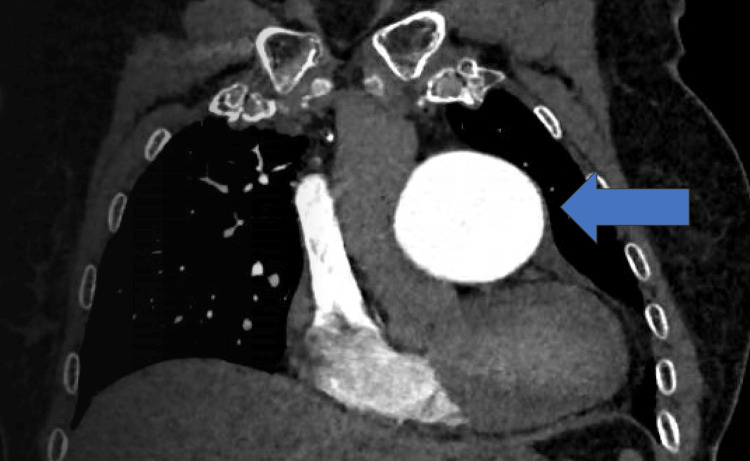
Coronal plane of pre-operative CT scan showing a giant PAA PAA: Pulmonary artery aneurysm
